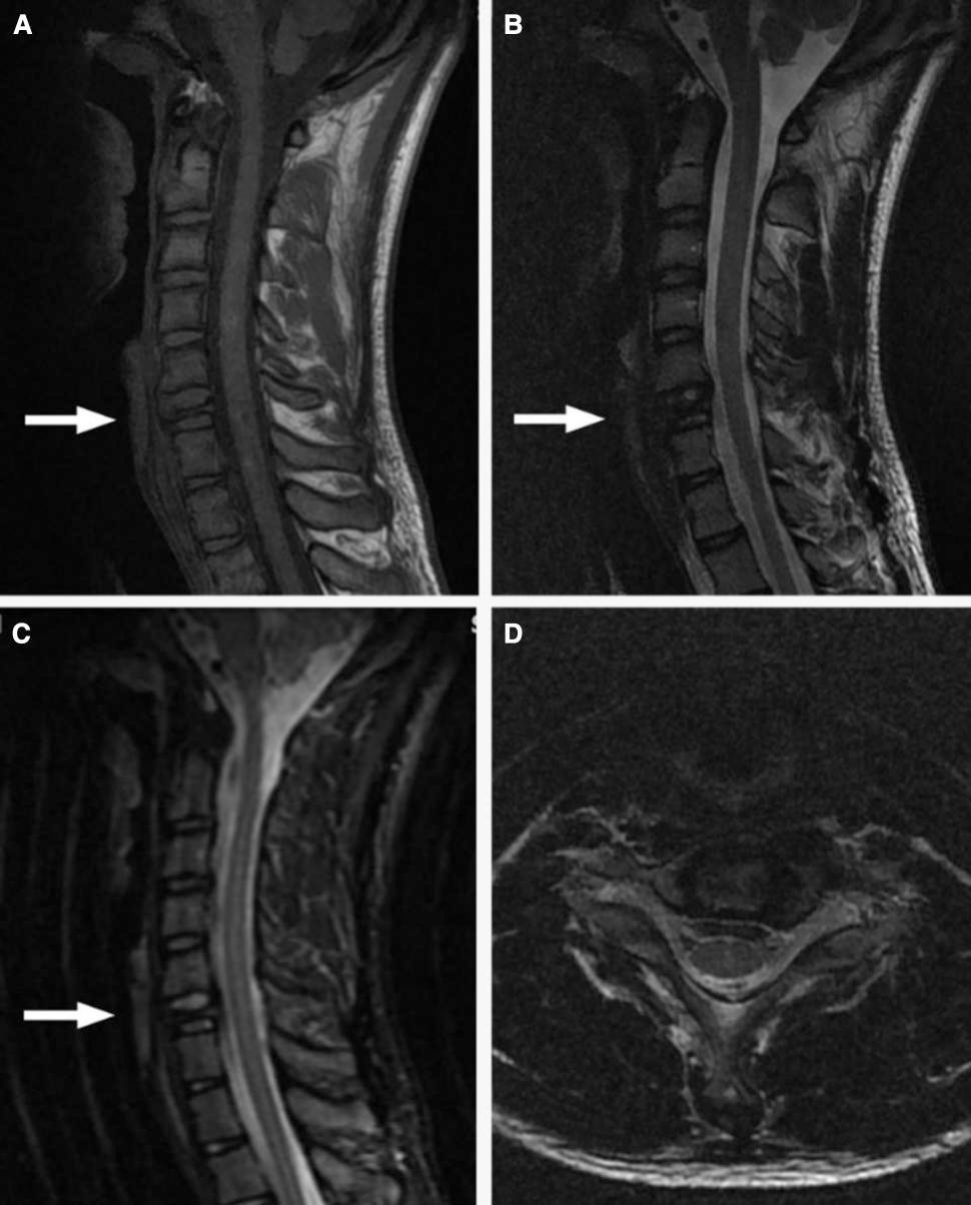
MRI of the cervical spine in multiple sequences and planes. Imaging demonstrates collapse of the C6 vertebra with approximately 90% loss of vertebral body height, consistent with vertebra plana, whereas the spinal cord remains unaffected. (A) Sagittal T1-weighted MR, (B) sagittal T2-weighted MRI, (C) sagittal short tau inversion recovery (STIR) MRI, and (D) axial T2-weighted MRI at the level of C6. In (A), (B), and (C), a white arrow indicates the level of the C6 vertebral body.
